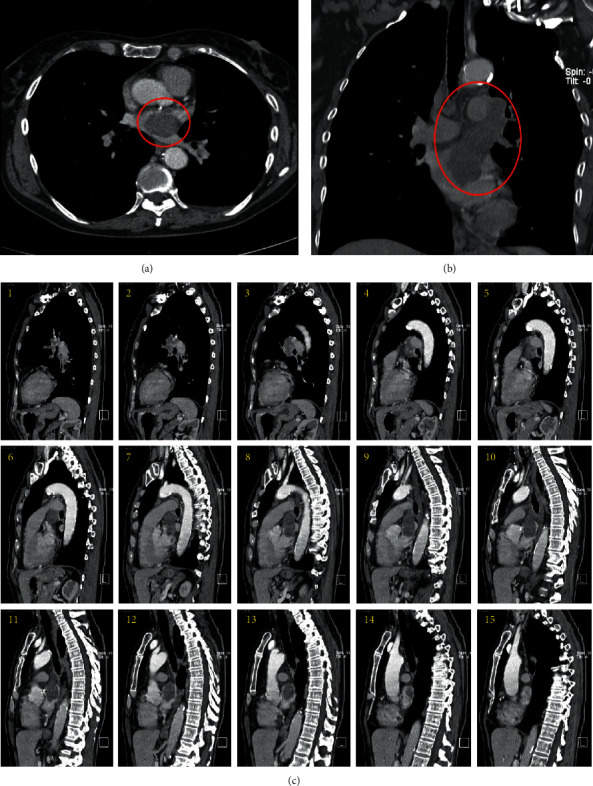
CT thorax with contrast sagittal (a) and coronal (b) representative views of the patient's mass measuring 3.3 × 2.4 cm. A sequence depicting the origination of the mass as it invades the left superior pulmonary vein before its extension in the left atrium (c).
